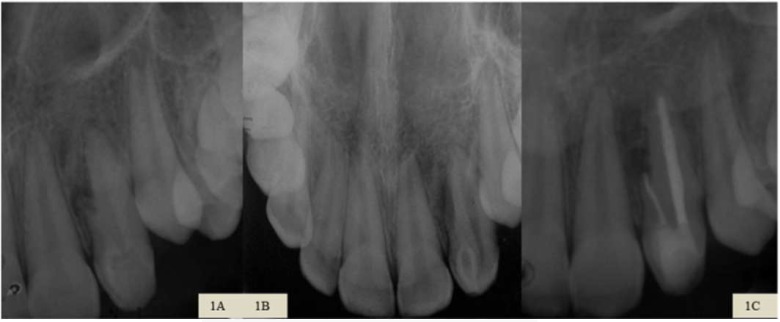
A)* Initial periapical radiography; *B)* Initial occlusal radiograph for verification of dens invaginatus in tooth 22 associated with lateral bone resorption; *C)* Final periapical radiography with root canal filling and restoration in tooth 22
